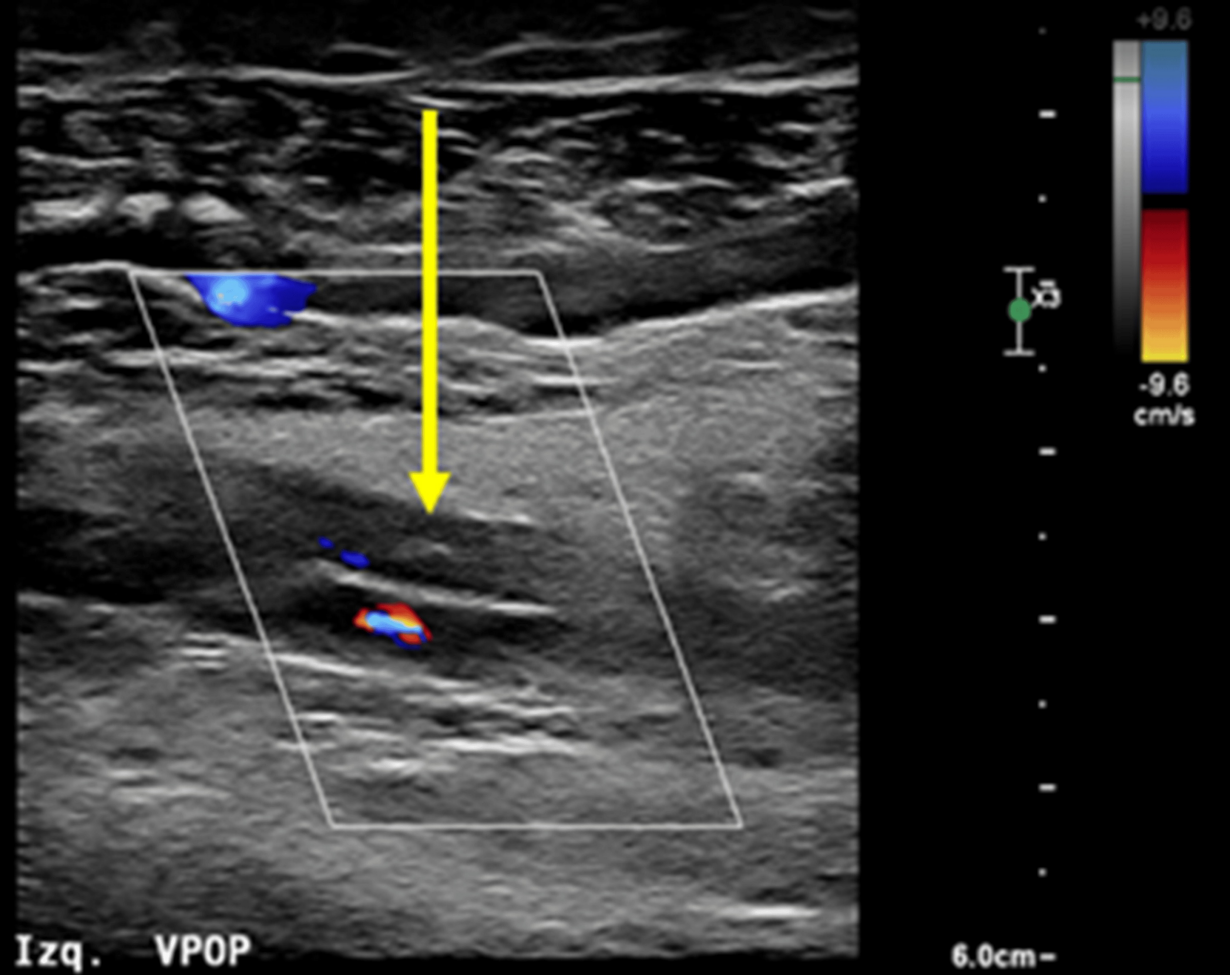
Lower limb ultrasound The arrow indicates a hyperechogenic thrombus within the left popliteal vein. Duplex Doppler examination confirms the absence of flow.
